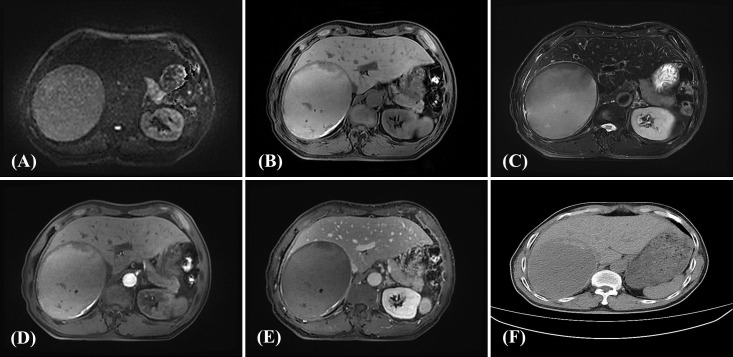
DWI demonstrated no appreciable restricted diffusion (A). The lesion appeared isointense to mildly hyperintense on T1-weighted imaging (T1WI) (B). The lesion demonstrated hyperintense signal on T2-weighted fat-suppressed imaging (FS-T2WI) (C). No appreciable enhancement was observed during the arterial phase of contrast-enhanced scanning (D). No appreciable enhancement was observed during the portal venous phase of contrast-enhanced scanning (E). CT imaging demonstrated a mildly hypodense mass in the posterior segment of the right hepatic lobe (F).
